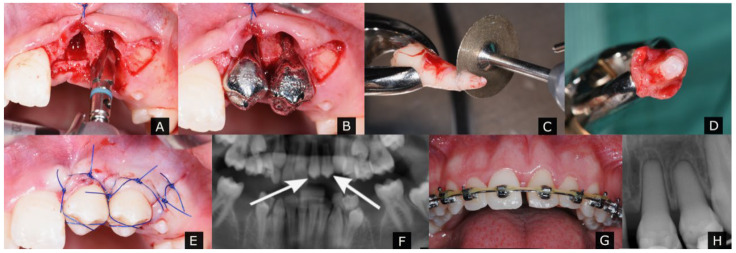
Autotransplantation of two mature premolars to the anterior left maxilla. (A) Recipient site preparation. (B) Tooth replica. (C) Root-end resection. (D) Enlarged diameter of the apical foramen. (E) Postoperative clinical image. (F) Postoperative radiograph image with arrows ranging from the donor to the recipient regions. (G) 4-year follow-up. (H) Intraoral radiograph 4 years after surgery with pulp obliteration.
